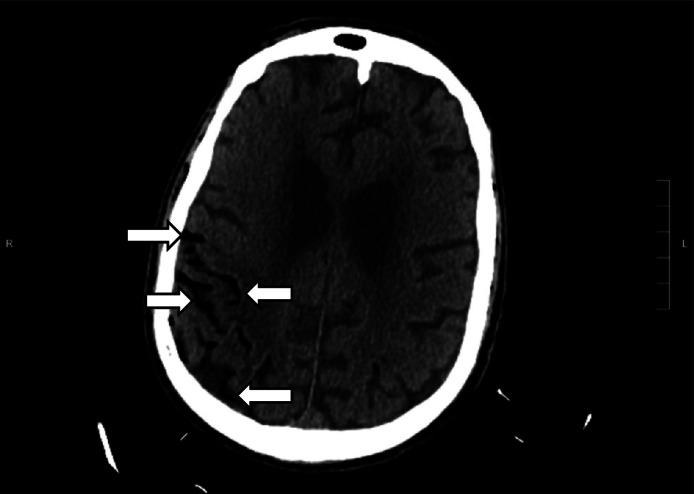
Computed tomography of the brain of a 77-year-old man after a rapid change in mental status showing extensive venous gas (white arrows) in the right parieto-occipital region.
